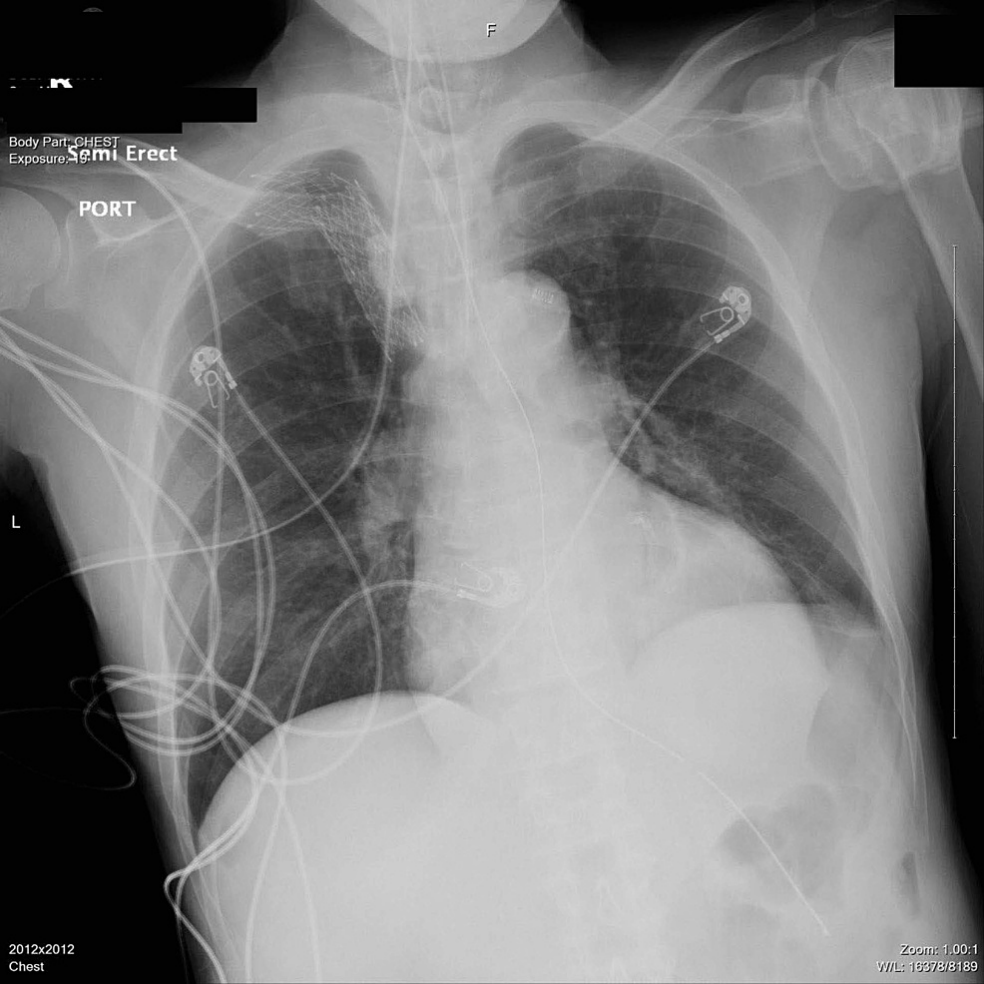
Chest X-ray revealed the resolution of infiltrates compared to the previous X-ray.
